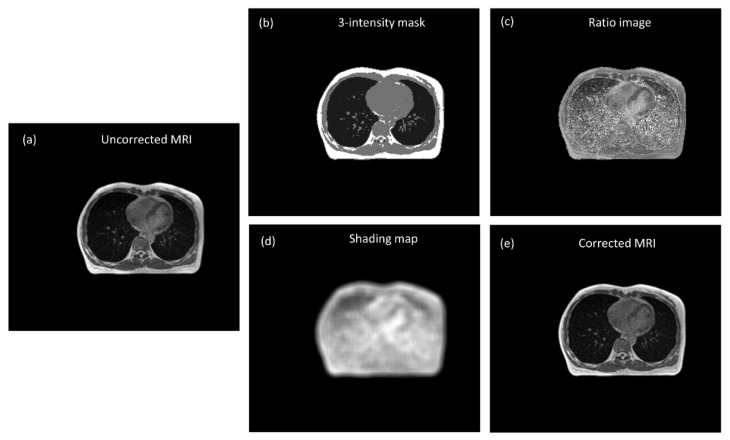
(a) Uncorrected MRI (WL/WW = 200/400), (b) three-intensity mask, (c) ratio image, (d) shading map, and (e) corrected MRI (WL/WW = 100/200).
